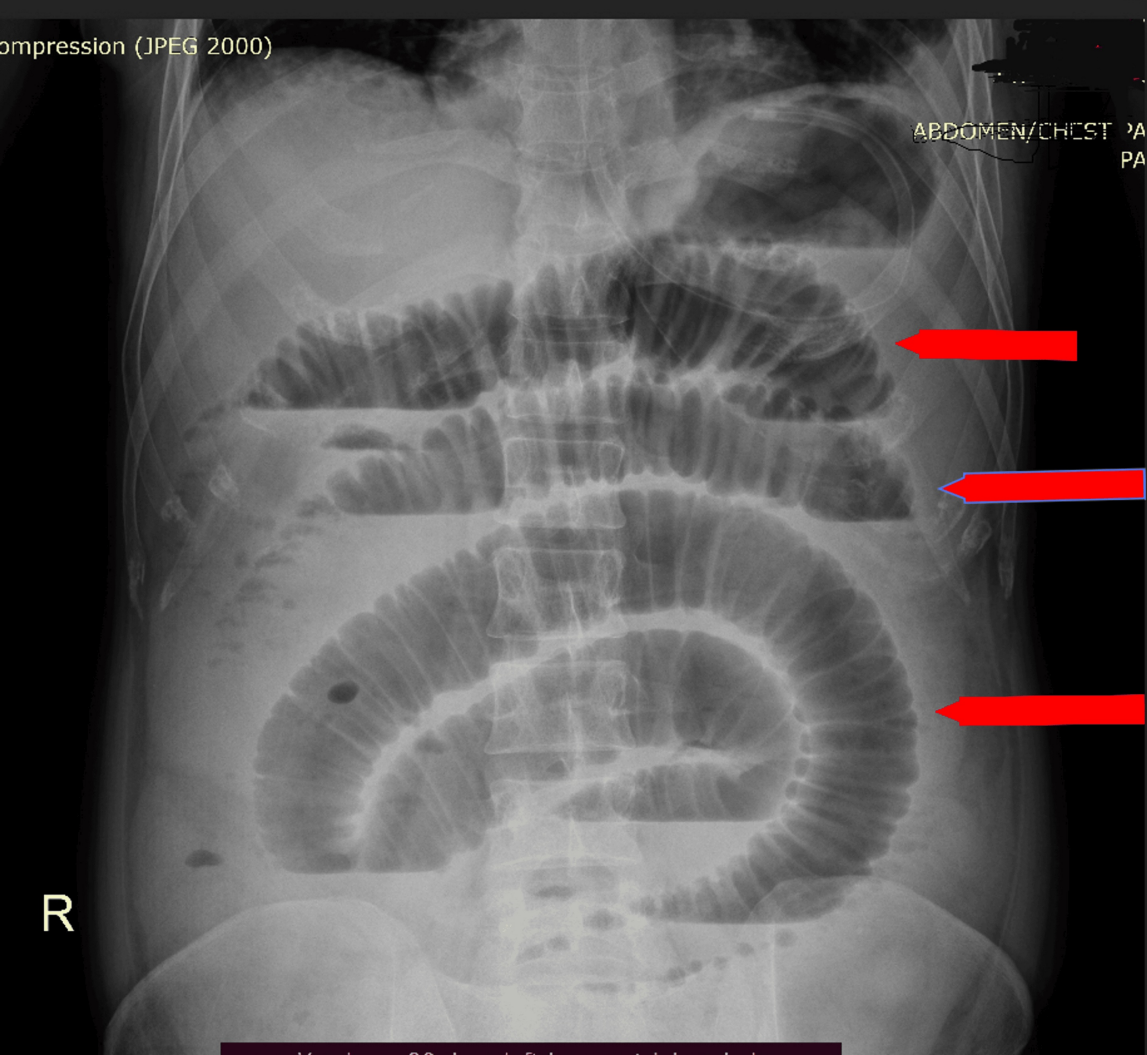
X-ray abdomen with arrows pointing at small bowel dilated air fluid levels.
